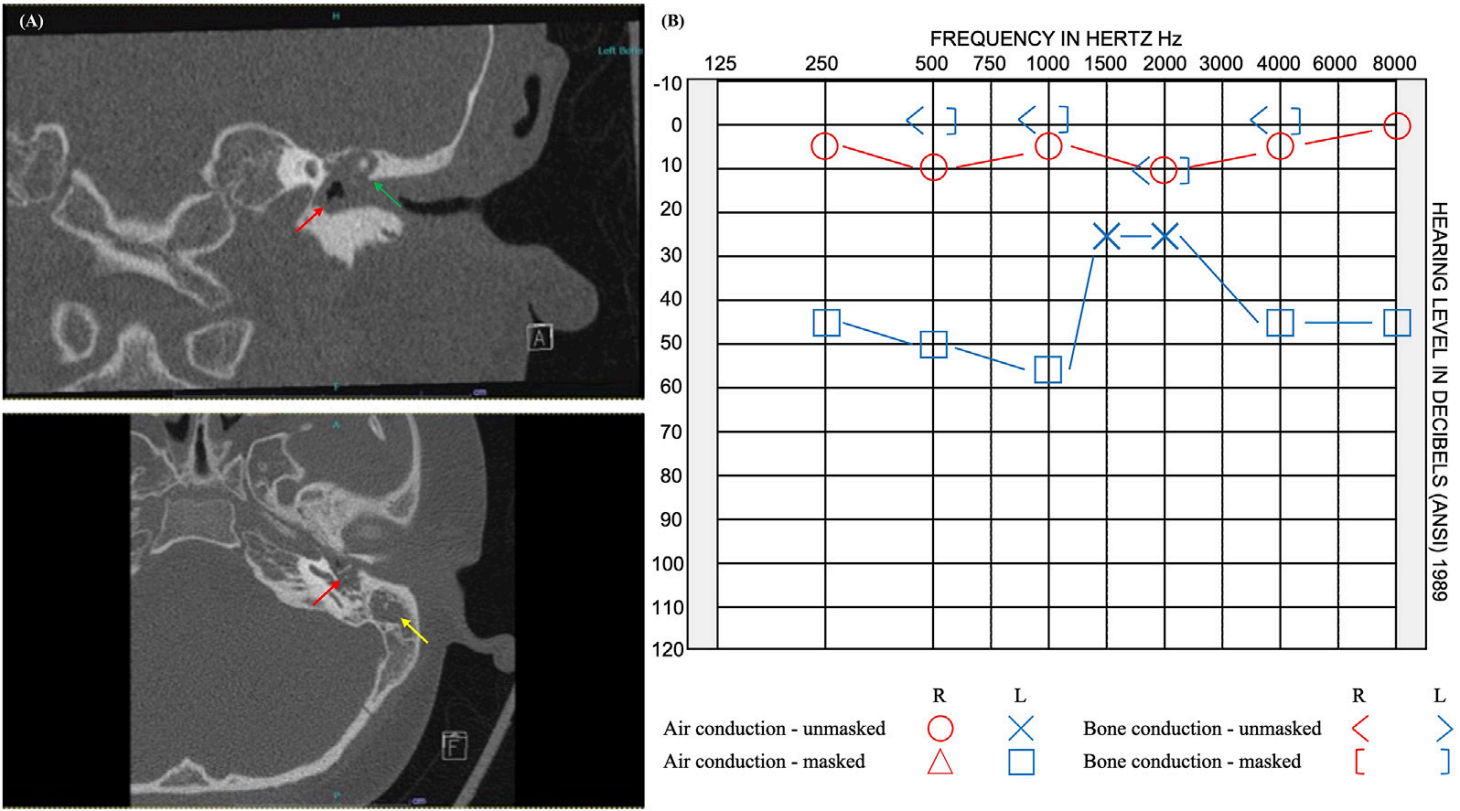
Imaging and audiogram results of pediatric patient with rare variants in 12 candidate genes. (A) CT imaging reveals opacification of the left middle ear cavity (red arrow), blunting of the scutum (green arrow), and complete opacification of the left mastoid (yellow arrow). (B) Audiogram results showed normal hearing at 250–8,000 Hz on the right and moderate rising to mild conductive hearing loss at 250–8,000 Hz on the left.
